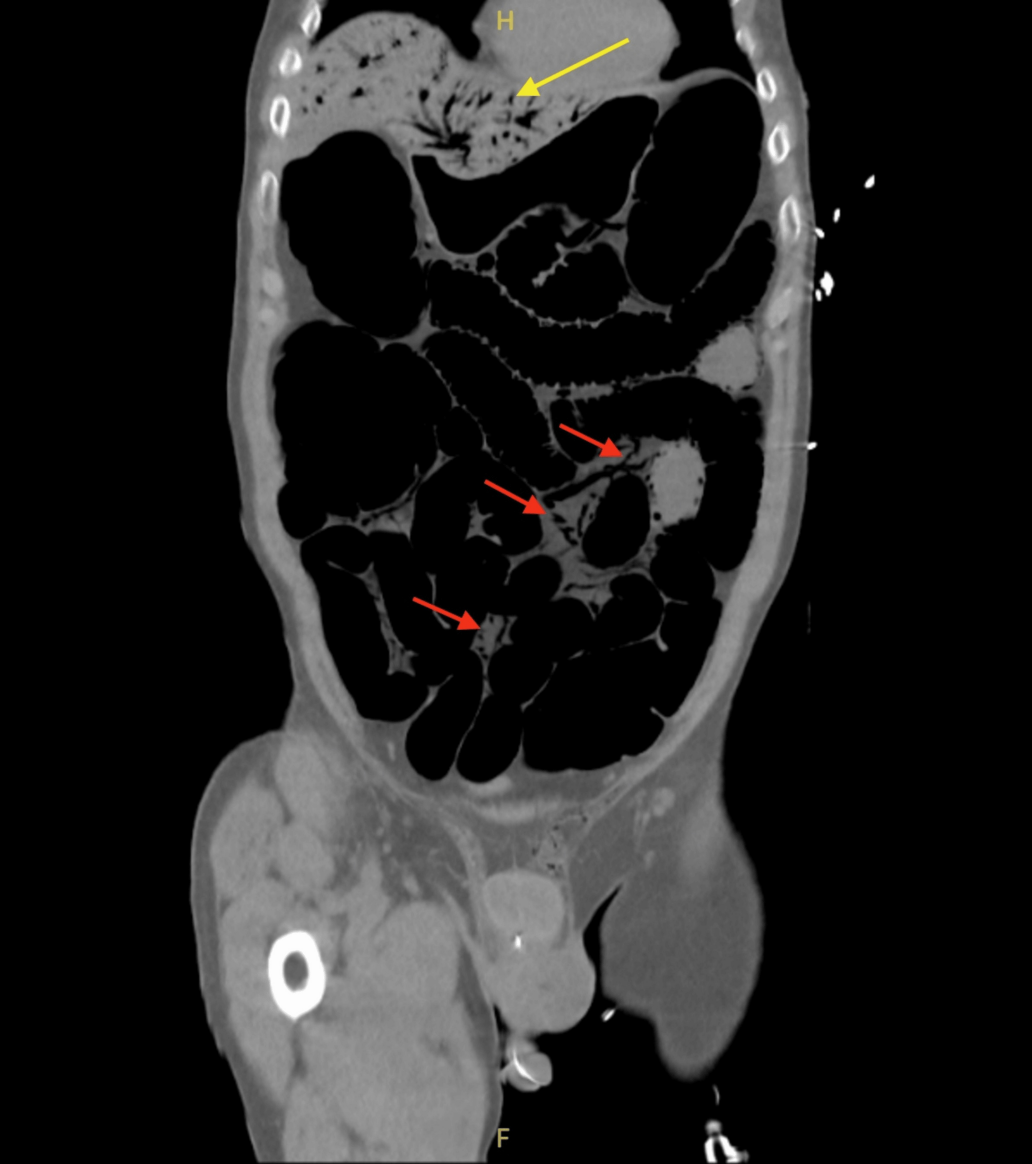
Coronal CT scan of the abdomen and pelvis without contrast demonstrating pneumatosis in the liver (yellow arrow), diffuse bowel distention, pneumatosis of the bowel (red arrows), and portal venous gas (yellow arrow)
